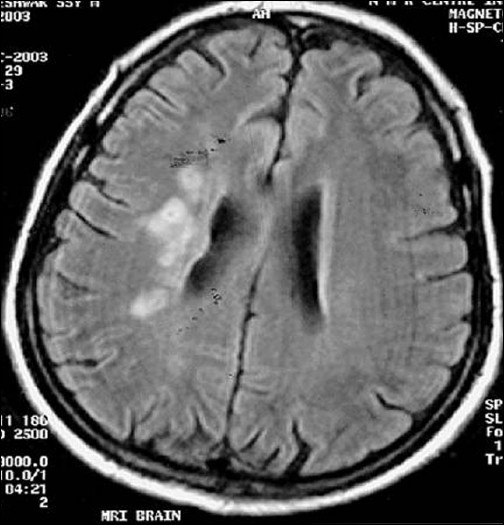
Second follow-up study shows progression of the same lesion on FLAIR image (second follow-up study of the same patient done 90 days after acute onset)
